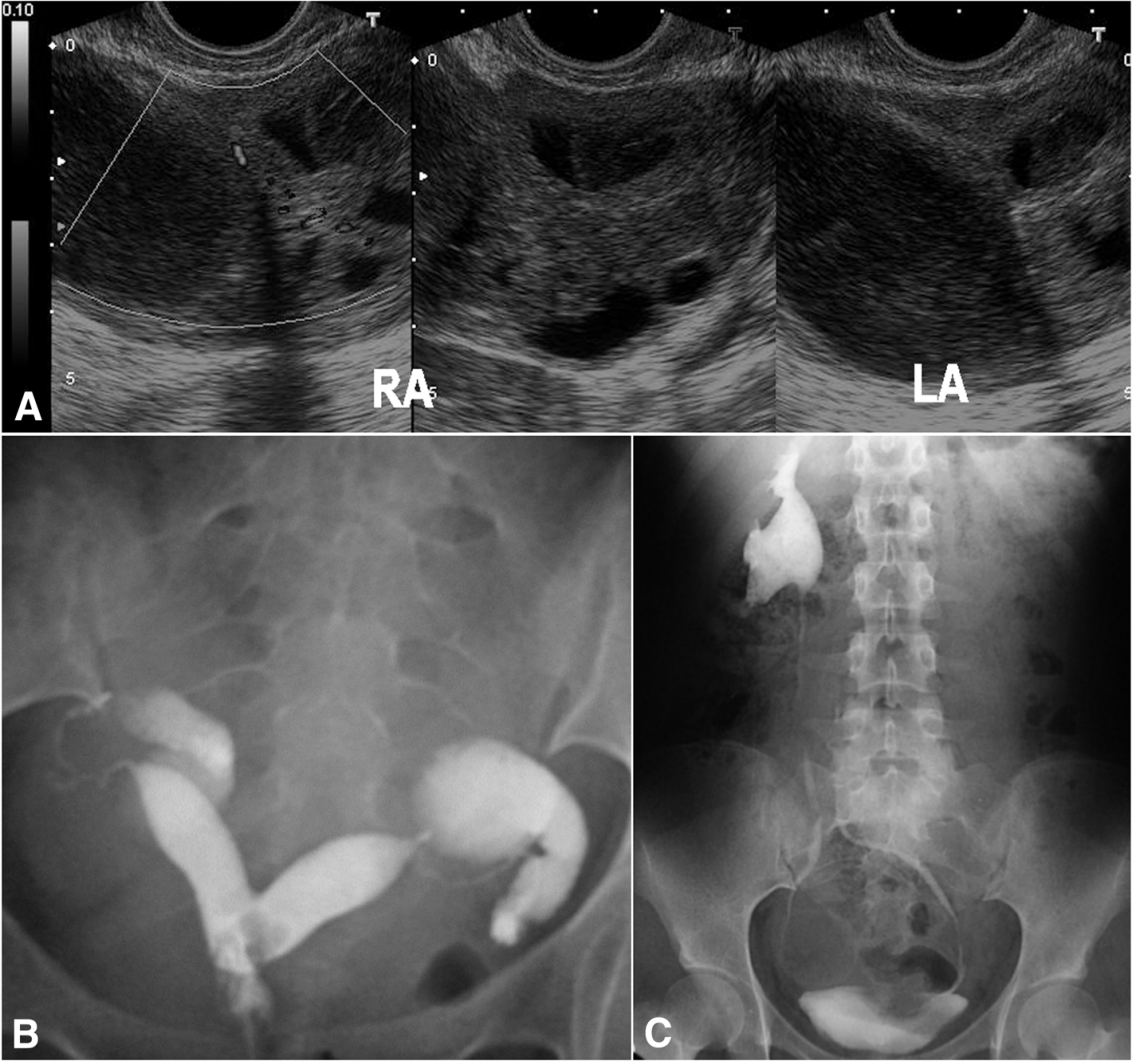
Diagnostic imaging tests. A. Transvaginal ultrasound findings; RA, right adnexum; LA, left adnexum. B. Hysterosalpingographic image showing bicornuate or subseptate uterus and bilateral hydrosalpinx. C. Intravenous pyelography showing the left pelvic kidney at the height of the promontory.
